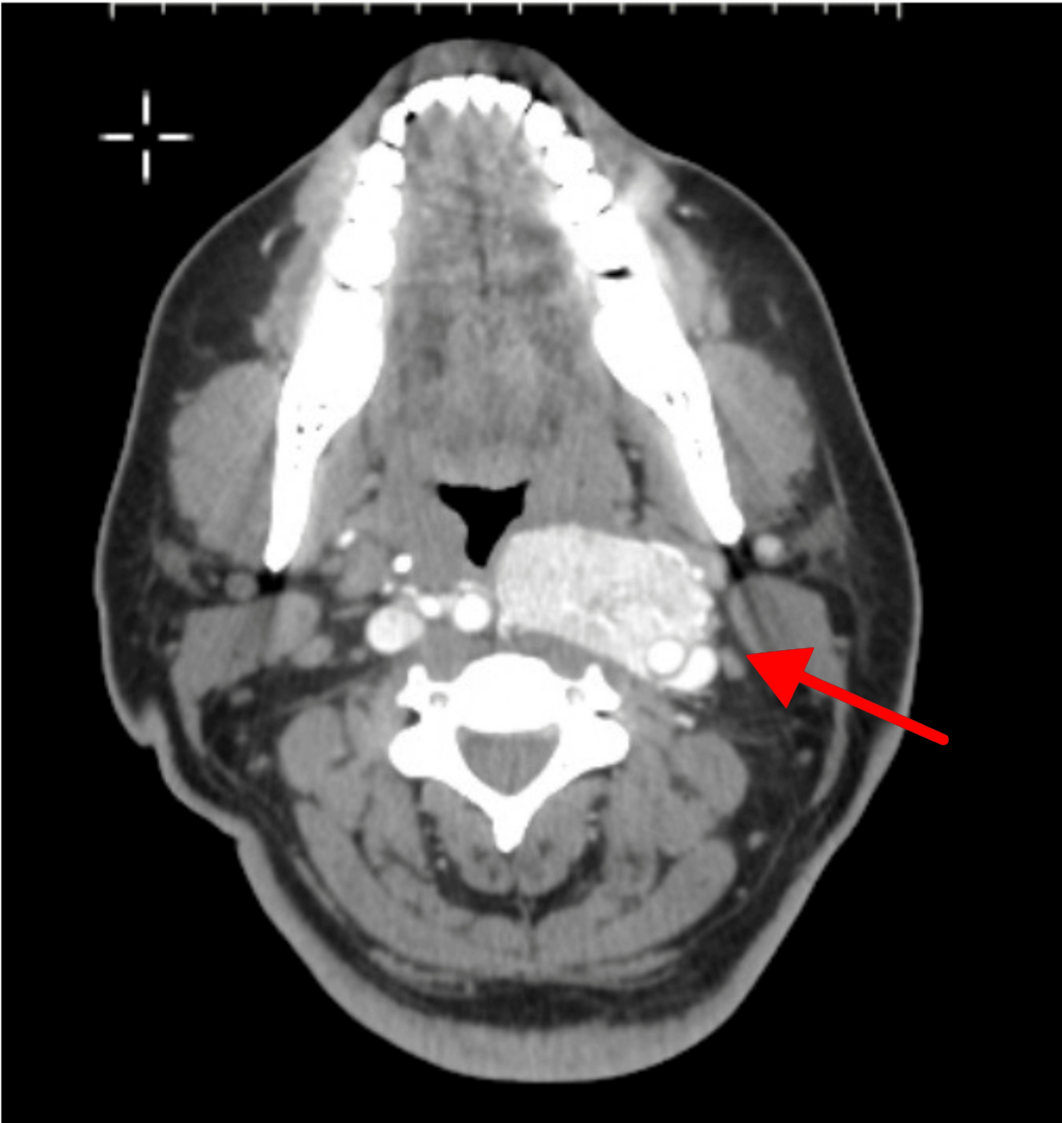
CT angiography showing an axial image of a CBT with tracheopharyngeal compression CBT, carotid body tumor
